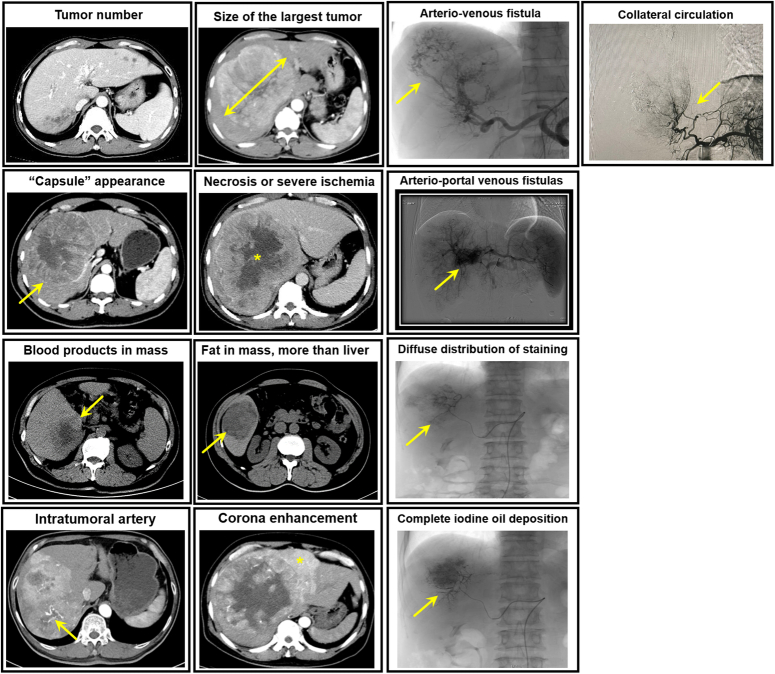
Examples of CT and digital subtraction angiography imaging features.
